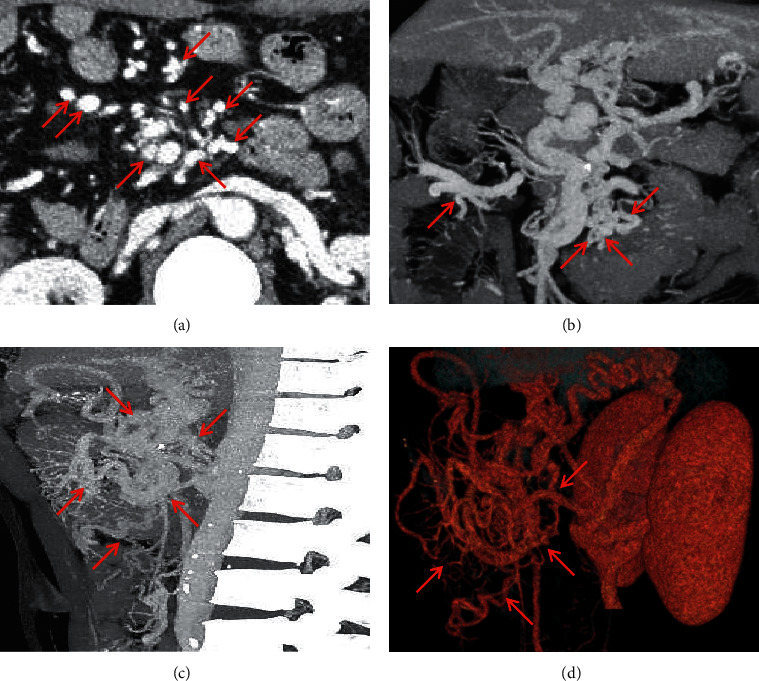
Retzius vein. A 48-year-old male patient with an eleven-year history of alcoholic-related liver cirrhosis presented with hematochezia. The contrast-enhanced CT scan on the portal vein phase demonstrated that the Retzius vein (red arrow) manifested as tortuously dilated vessels in the retroperitoneum in the portal vein phase. (a) Axial contrast-enhanced CT; (b) coronal contrast-enhanced CT; (c) sagittal contrast-enhanced CT; (d) three-dimensional reconstruction.
